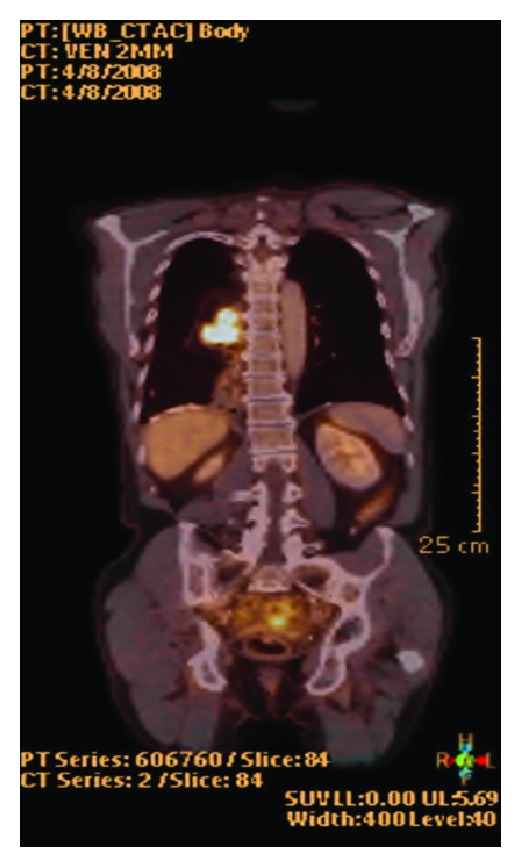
The PET-CT showed a tumor paravertebral right at the level of D7–D9 and no metastatic disease.
